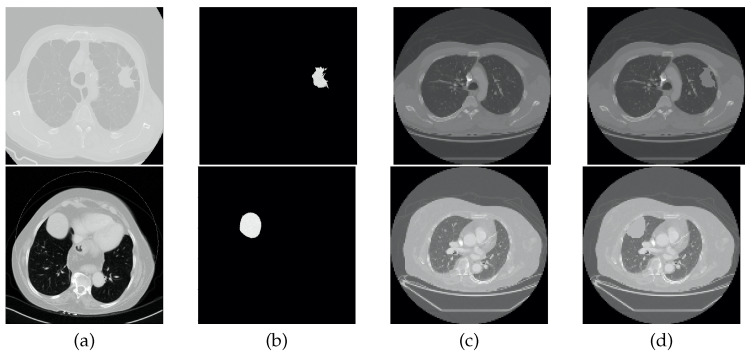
Example of data augmentation. From left to right: (a) original CT image containing a nodule; (b) ROI of the nodule; (c) original CT image without nodules; (d) and synthetic CT image that includes a nodule.
